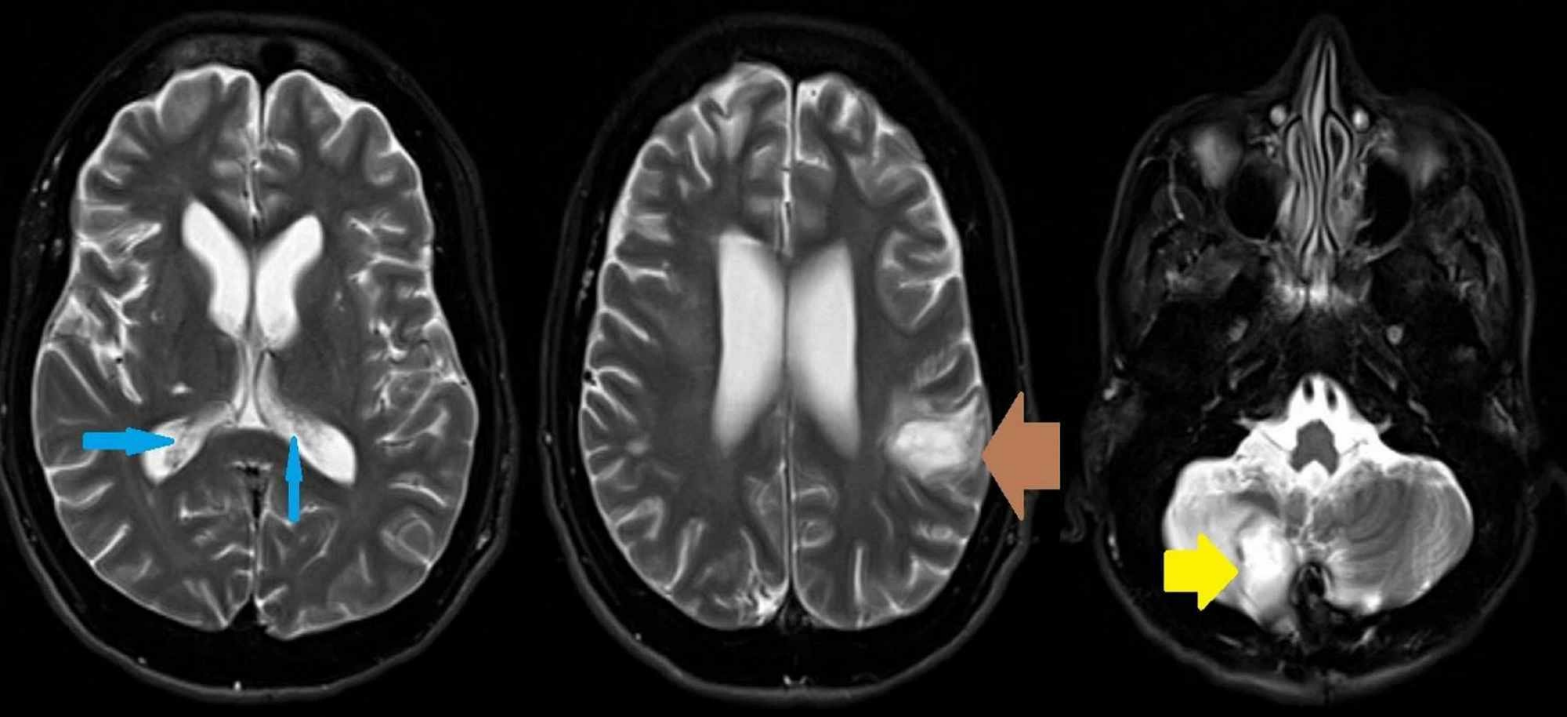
Magnetic resonance imaging of the brain showing ventriculitis (blue arrows), enlarged left parietal abscess (brown arrow), and right cerebellar abscess (yellow arrow)
